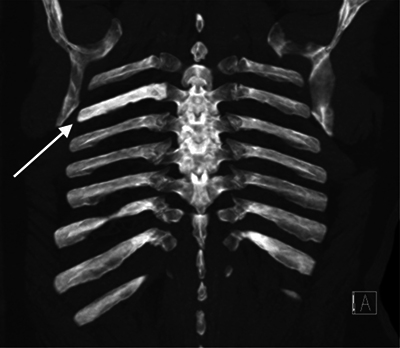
CT thorax coronal MIP images.
